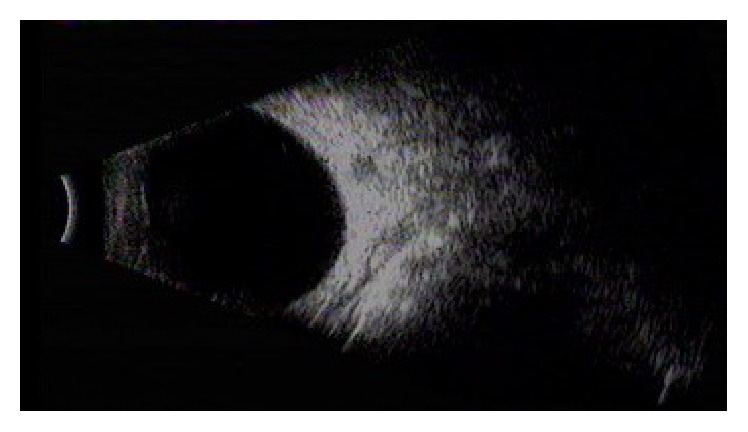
B-scan ultrasonography showed a clear vitreous cavity of a patient after the surgery of silicone oil removal.
